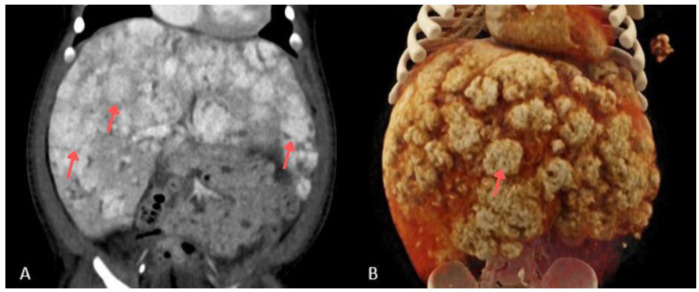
CT of the abdominal cavity with intravenous contrast administration in the venous phase, coronal section (A), and CT with Volume Rendering Technique (VRT) (B), performed during the first week of hospitalization. Some lesions were marked with arrows.
